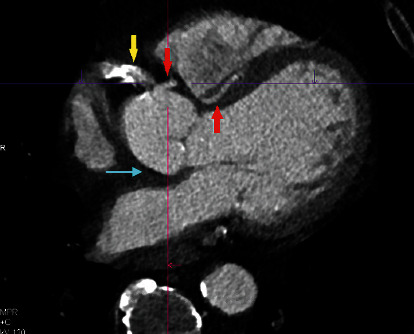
Coronary CT showed the absence of a left coronary system (blue arrow), a large calcified RCA originating posteriorly from the right coronary cusp (yellow arrow), and a small LAD artery originating from the right coronary cusp into the interventricular groove (red arrows).
